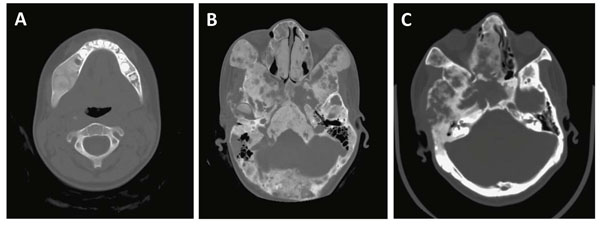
Variations in CT appearance of fibrous dysplasia based on age. A) FD in the young patient most often appears as homogenous, radio-dense lesions often described as having a ground glass appearance on CT. B) As these patients enter adolescence, the FD lesions progress to a mixed appearance which stabilizes in adulthood (C) but does not necessarily resume a homogenous appearance. This may explain the numerous radiographic descriptions of FD in the literature such as “ground-glass”, “pagetoid”, “lytic”, and “cystic”.
